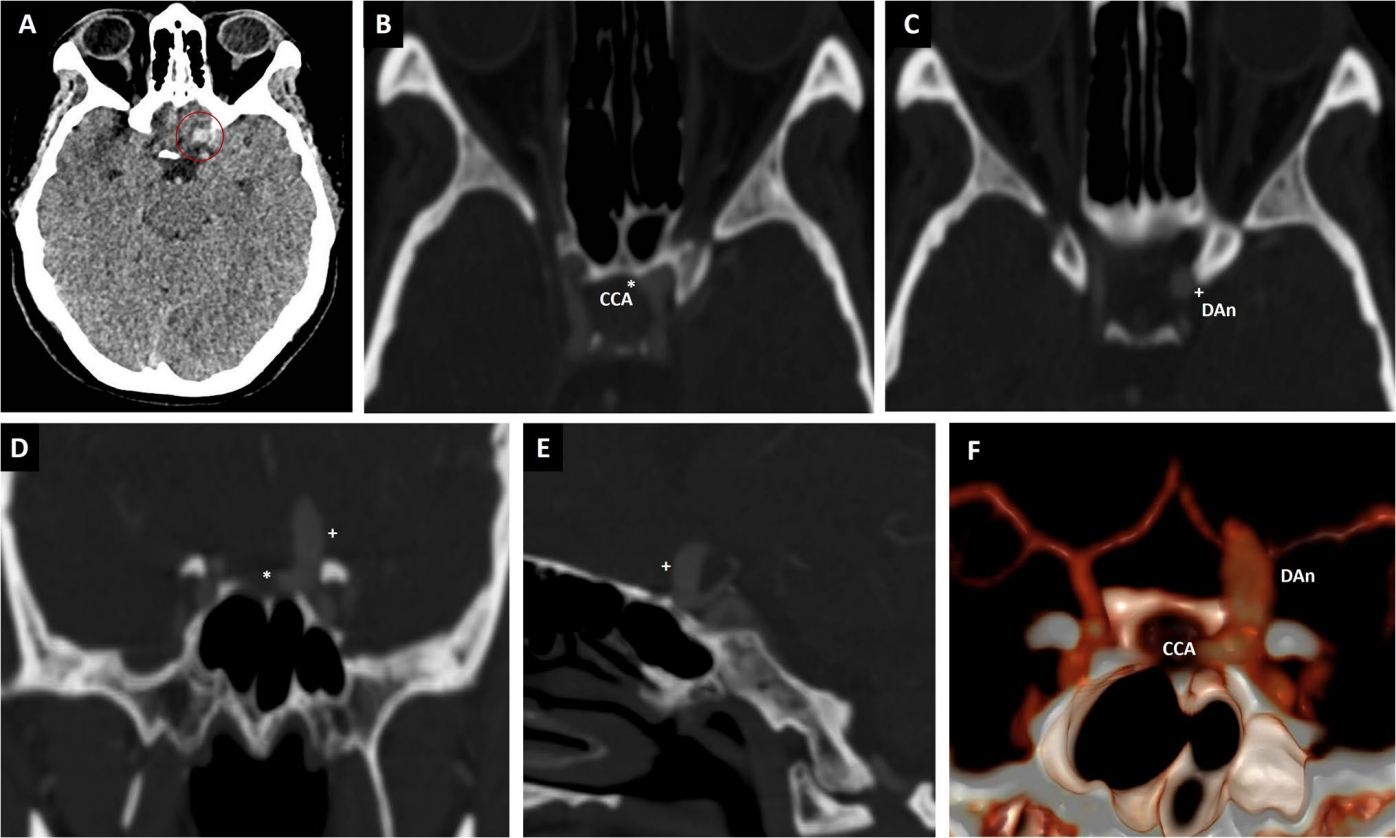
A previously healthy 43-year-old female, complaining of thunderclap headache 9 days before, was admitted to the emergency room with a 4/5 right hemiparesis. A CT scan showed a hyperdensity medial to the left ACP (red circle). B A CT angiography in the axial showing a carotid cave aneurysm (CCA), and C a dorsal paraclinoid aneurysm (DAn). Coronal (D), sagittal (E), windows show a 3 × 4-mm left carotid cave aneurysm (*) and a 5 × 11-mm left dorsal paraclinoid aneurysm ( +). F shows a CTA 3D reconstruction with both aneurysms closely related to the left ACP
